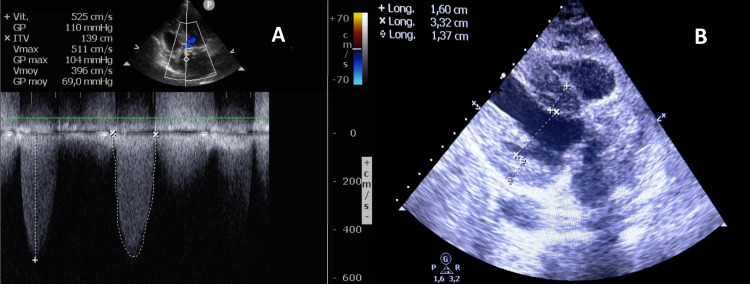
Preoperative transthoracic echocardiogram showing severe aortic stenosis A: Continuous-wave Doppler signal showing a transaortic mean gradient of 69 mmHg and a maximum velocity of 5.1 m/s. B: Asymmetrical left ventricular septal hypertrophy, measured at 16 mm.
